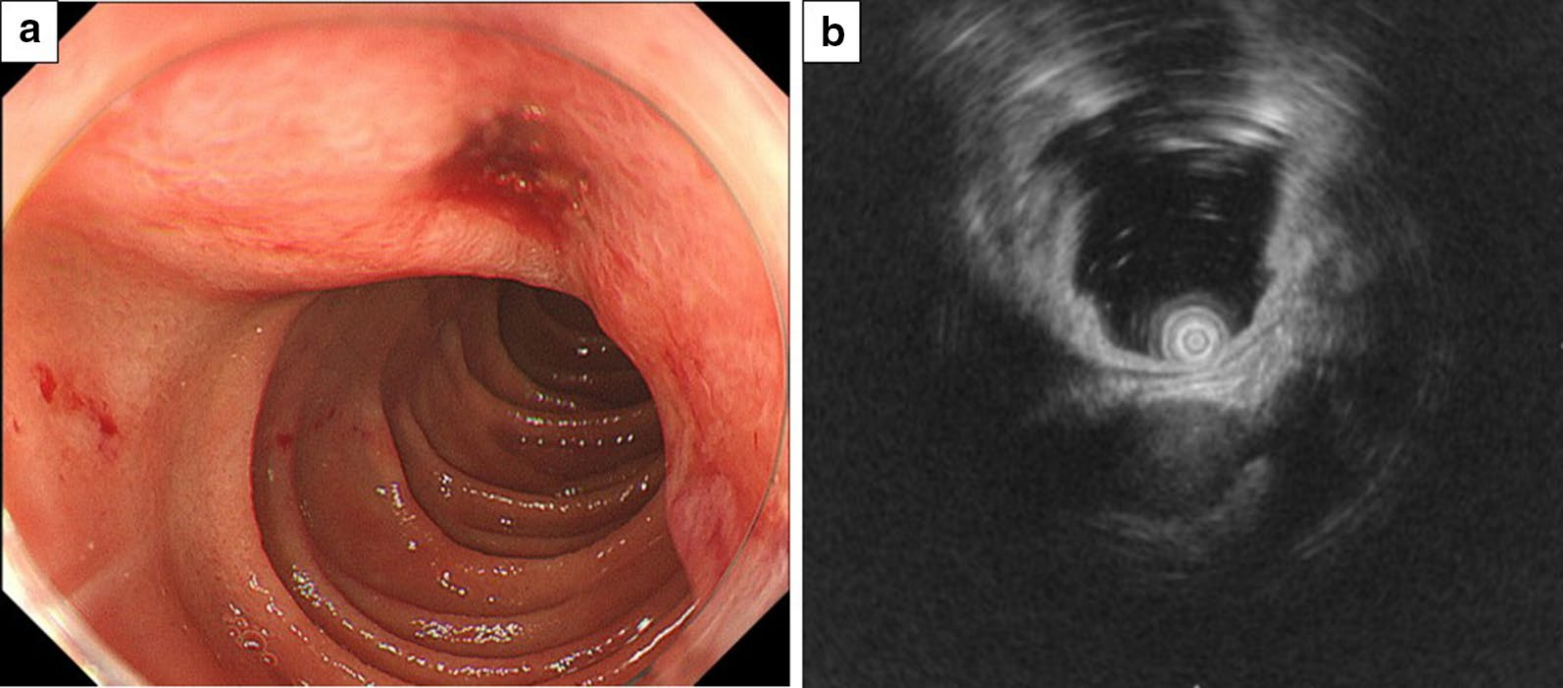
a Endoscopy shows a round, smooth, elevated mass in the second portion of the duodenum with central ulceration. b Endoscopic ultrasonography of the lesion confirms a hypoechoic mass arising from the fourth layer of the duodenal wall
